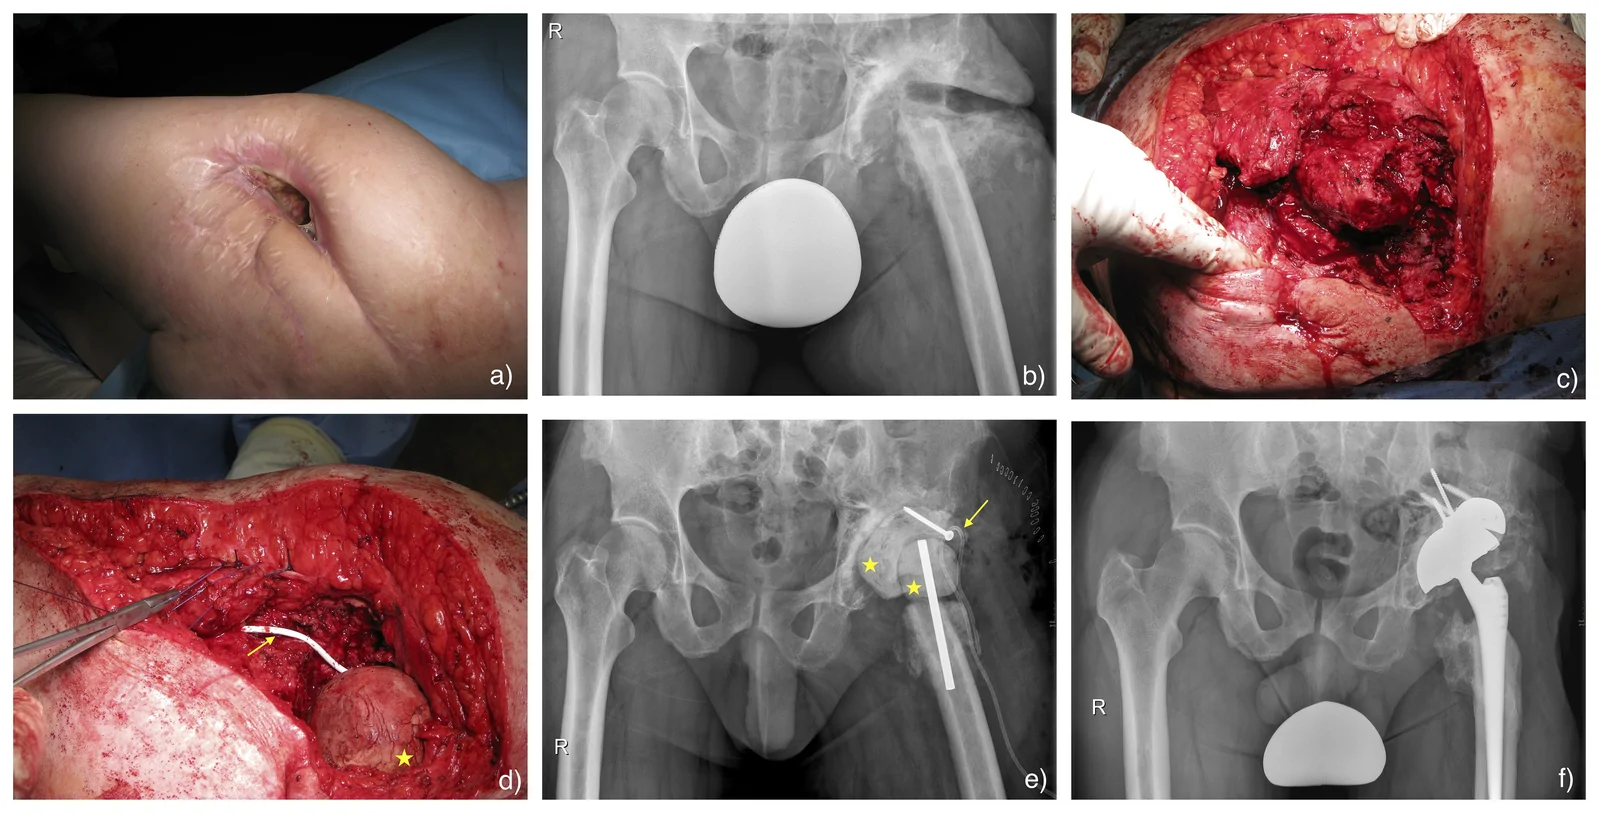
(a) Extensive cutaneous sinus tract on the lateral aspect of the left hip. (b) Radiograph at referral demonstrating heterotopic ossification and sinus tract communication to the acetabulum, following resection of the proximal femur and greater trochanter. (c) Intraoperative view of the exposed infection focus prior to debridement. (d) Intraoperative view following debridement, with an antibiotic-impregnated cement spacer (
